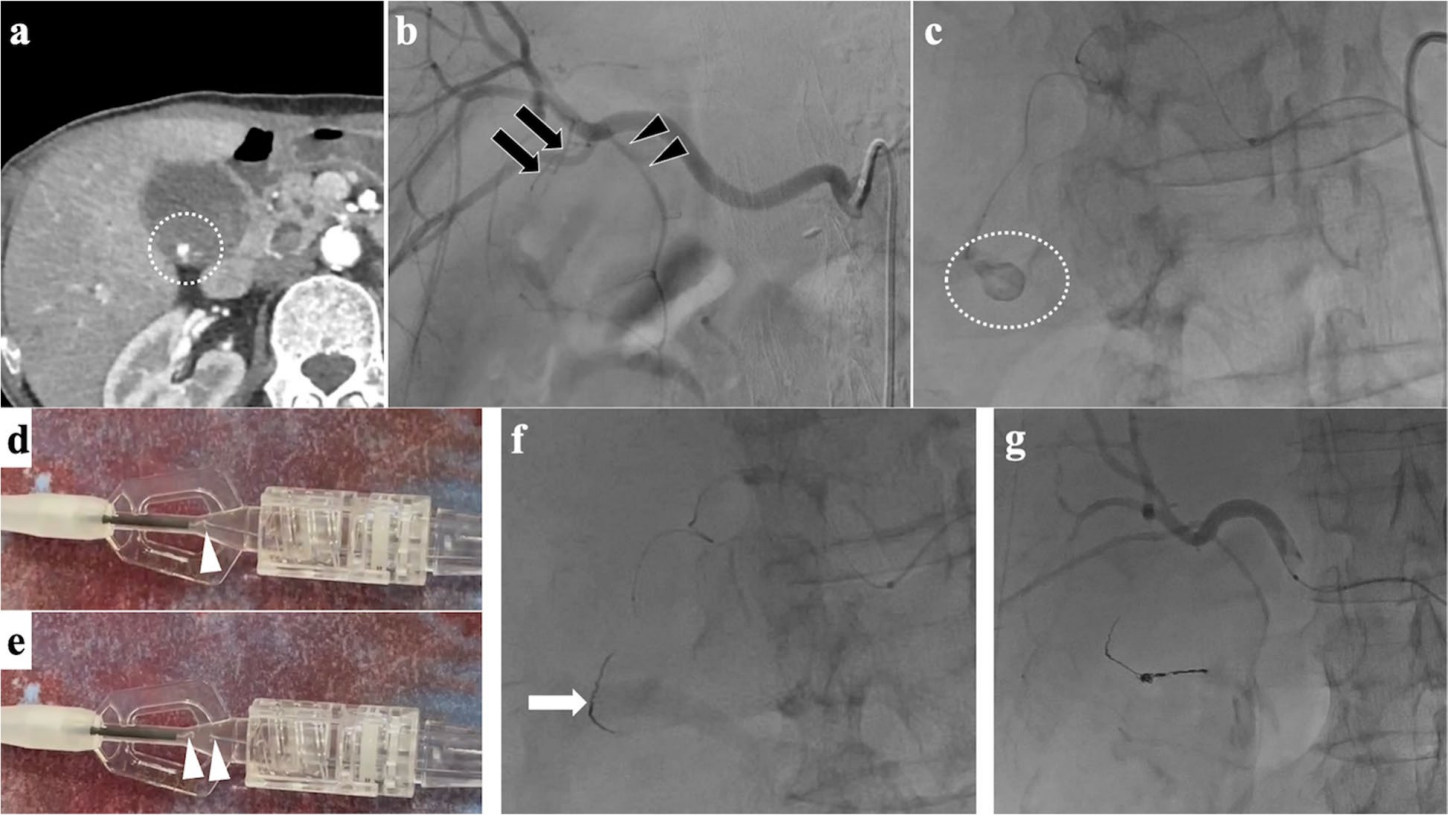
A 69-year-old male presented with hematemesis. a contrast-enhanced computed tomography demonstrated pseudoaneurysm in the gallbladder (circle); b angiography from the replaced right hepatic artery revealed two cystic arteries (black arrow and arrowhead); c the selection of the right-sided cystic artery (black arrow) was difficult, and a 1.3-Fr microcatheter (DeFrictor Nano; Medico's Hirata, Osaka, Japan) was used. Superselective angiography through the cystic artery showed a pseudoaneurysm (circle); d and e advancing just the coil section of the 0.010-inch Target Nano coil (Stryker, Fremont, CA, USA), which measures 1 mm in diameter and 3 cm in length, into the microcatheter and ensuring that the detachment point is slightly within the catheter hub (white arrowhead), allows for successful detachment; f two 0.010-inch coils were placed (white arrow) using the intentional early detachment technique. The left-sided cystic artery was easily selected with a 1.6-Fr catheter (Carnelian MARVEL S; Tokai Medical Products, Aichi, Japan) and embolized with three detachable coils using the standard method; g angiography after embolization revealed the complete embolization of the pseudoaneurysm
